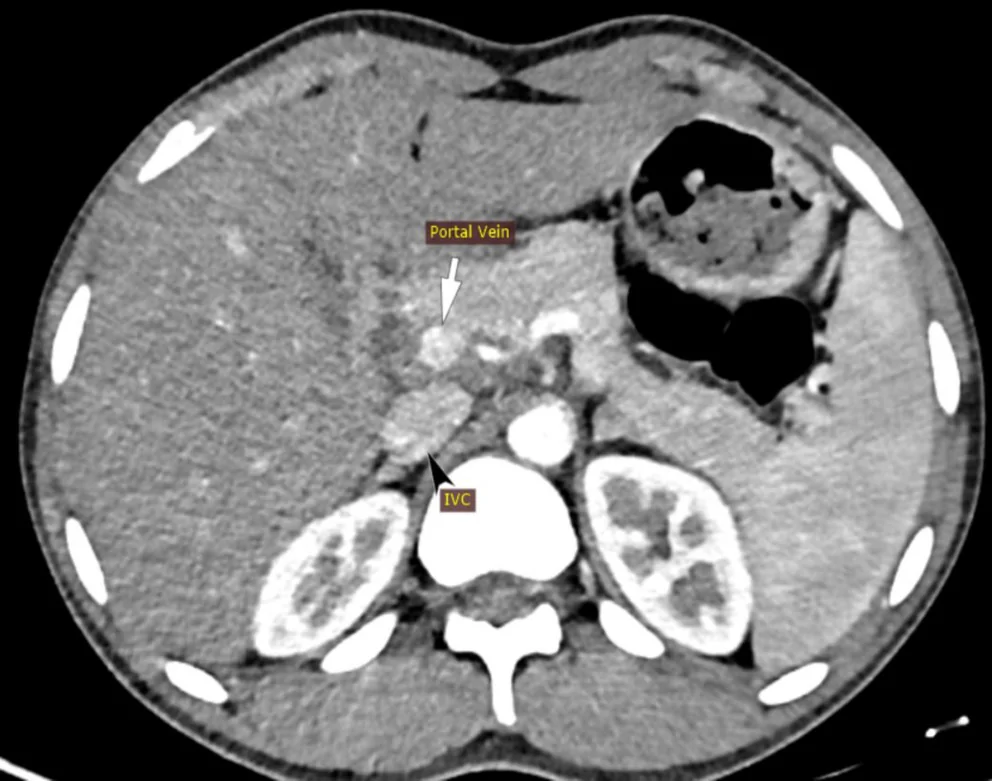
Axial contrast‐enhanced CT image at the level of the porta hepatis, obtained 10 weeks after treatment initiation, demonstrating complete resolution of the periportal lesion and marked improvement of the intrahepatic biliary dilatation.
